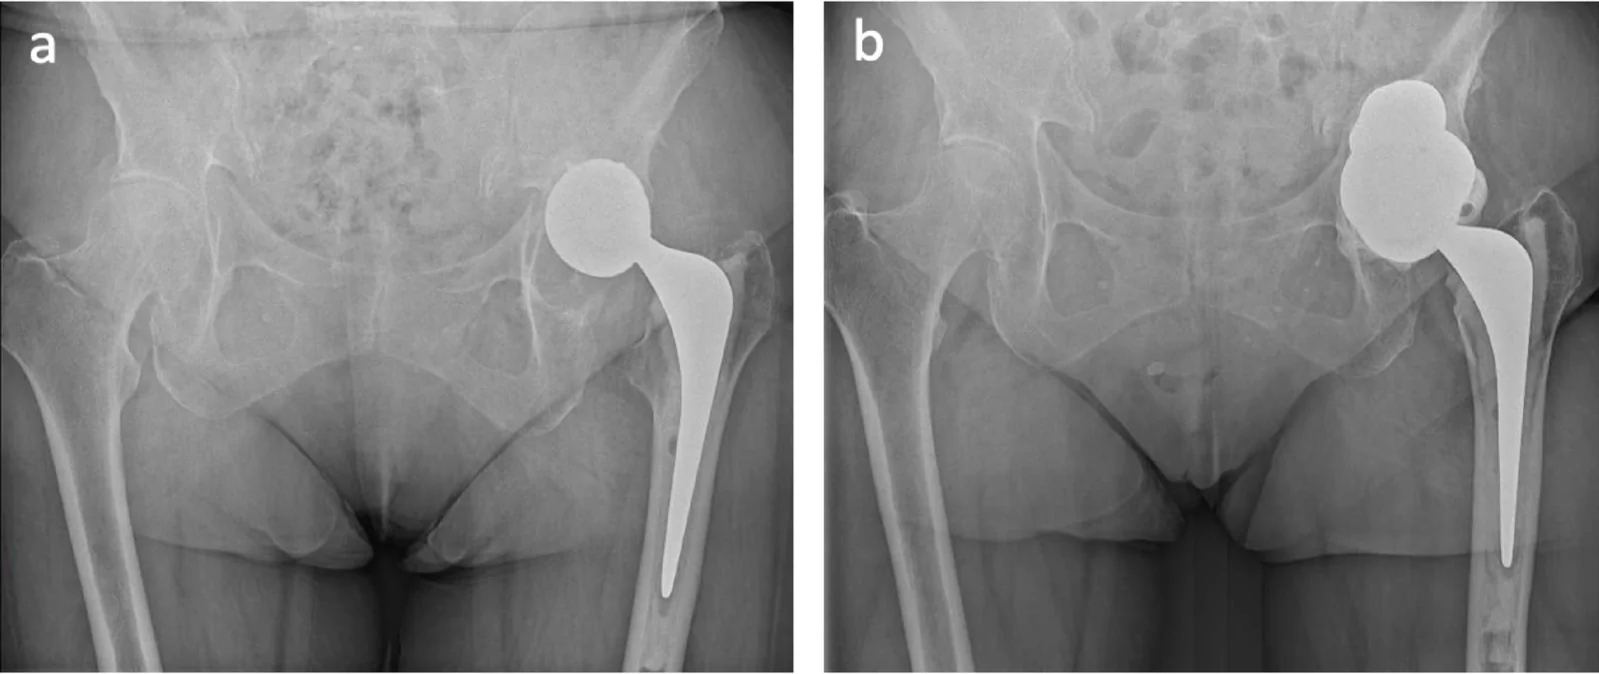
Anteroposterior pelvis radiographs demonstrating revision of a failed acetabular component with structural bone deficiency. a Preoperative view showing the failed construct. b Postoperative view following reconstruction using a porous revision shell with structural augments to optimize bone contact, into which a dual-mobility monoblock cup was cemented to independently control component orientation
